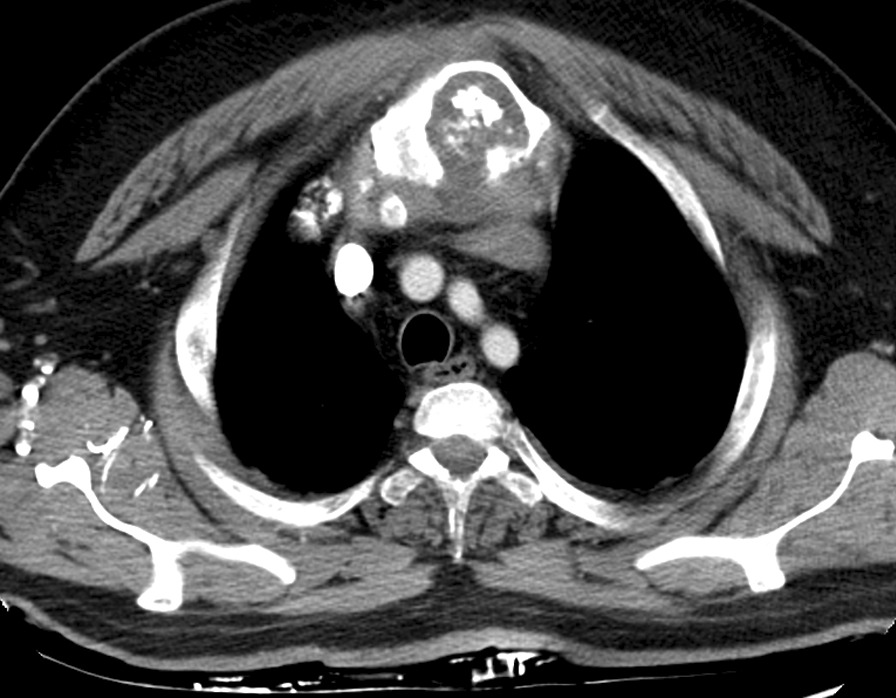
Pre-operative computed tomography (CT) of anterior chest wall chondrosarcoma arising from the sternum with internal calcification
